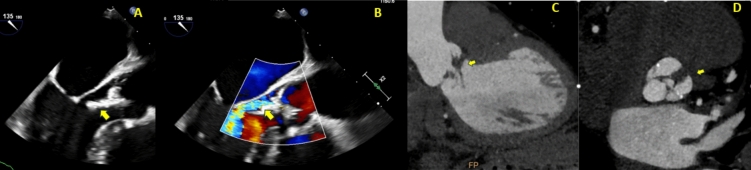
61-year-old gentleman presenting with subacute infective endocarditis. He had a three-week history of night sweats, chills, and rigors, along with a three-to-six-month history of exertional dyspnea and chest tightness. Transesophageal echocardiogram revealed thickening of the aortic valve, mobile vegetations, and moderate aortic regurgitation. A CT coronary angiogram, performed as part of his surgical workup, confirmed thickening of the aortic valve leaflets, a central coaptation defect. *Panel A* shows an abnormal echodensity attached to the aortic valve. *Panel B* shows the aortic regurgitation jet on long-axis images of the transesophageal echocardiogram. *Panel C* shows the filamentous density attached to the thickened aortic valve on CT. *Panel D* reveals the thickened bicuspid aortic valve with a raphe between the left and right coronary cusps and central malcoaptation as the site of the patient's aortic regurgitation
